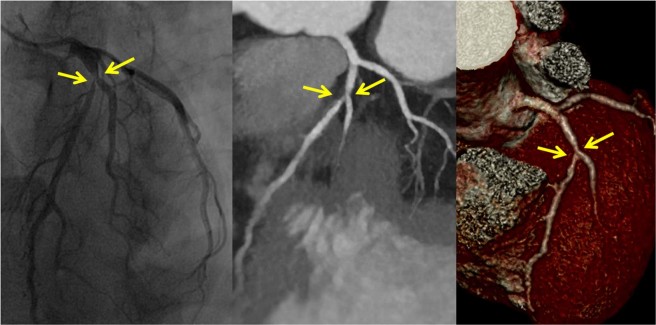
True positive finding with left anterior descending stenosis. Proximal left anterior descending stenosis in a 53-year-old woman (effective radiation dose, 0.53 mSv; BMI, 20.3 kg/m^2^; mean heart rate, 60 bpm), diagnosed via invasive coronary angiography (a), and examined via coronary computed tomographic angiography under 80 kVp/100 mAs, with maximum intensity projection (b) and volume-rendered (c) images and iterative model reconstruction algorithm reconstruction.
